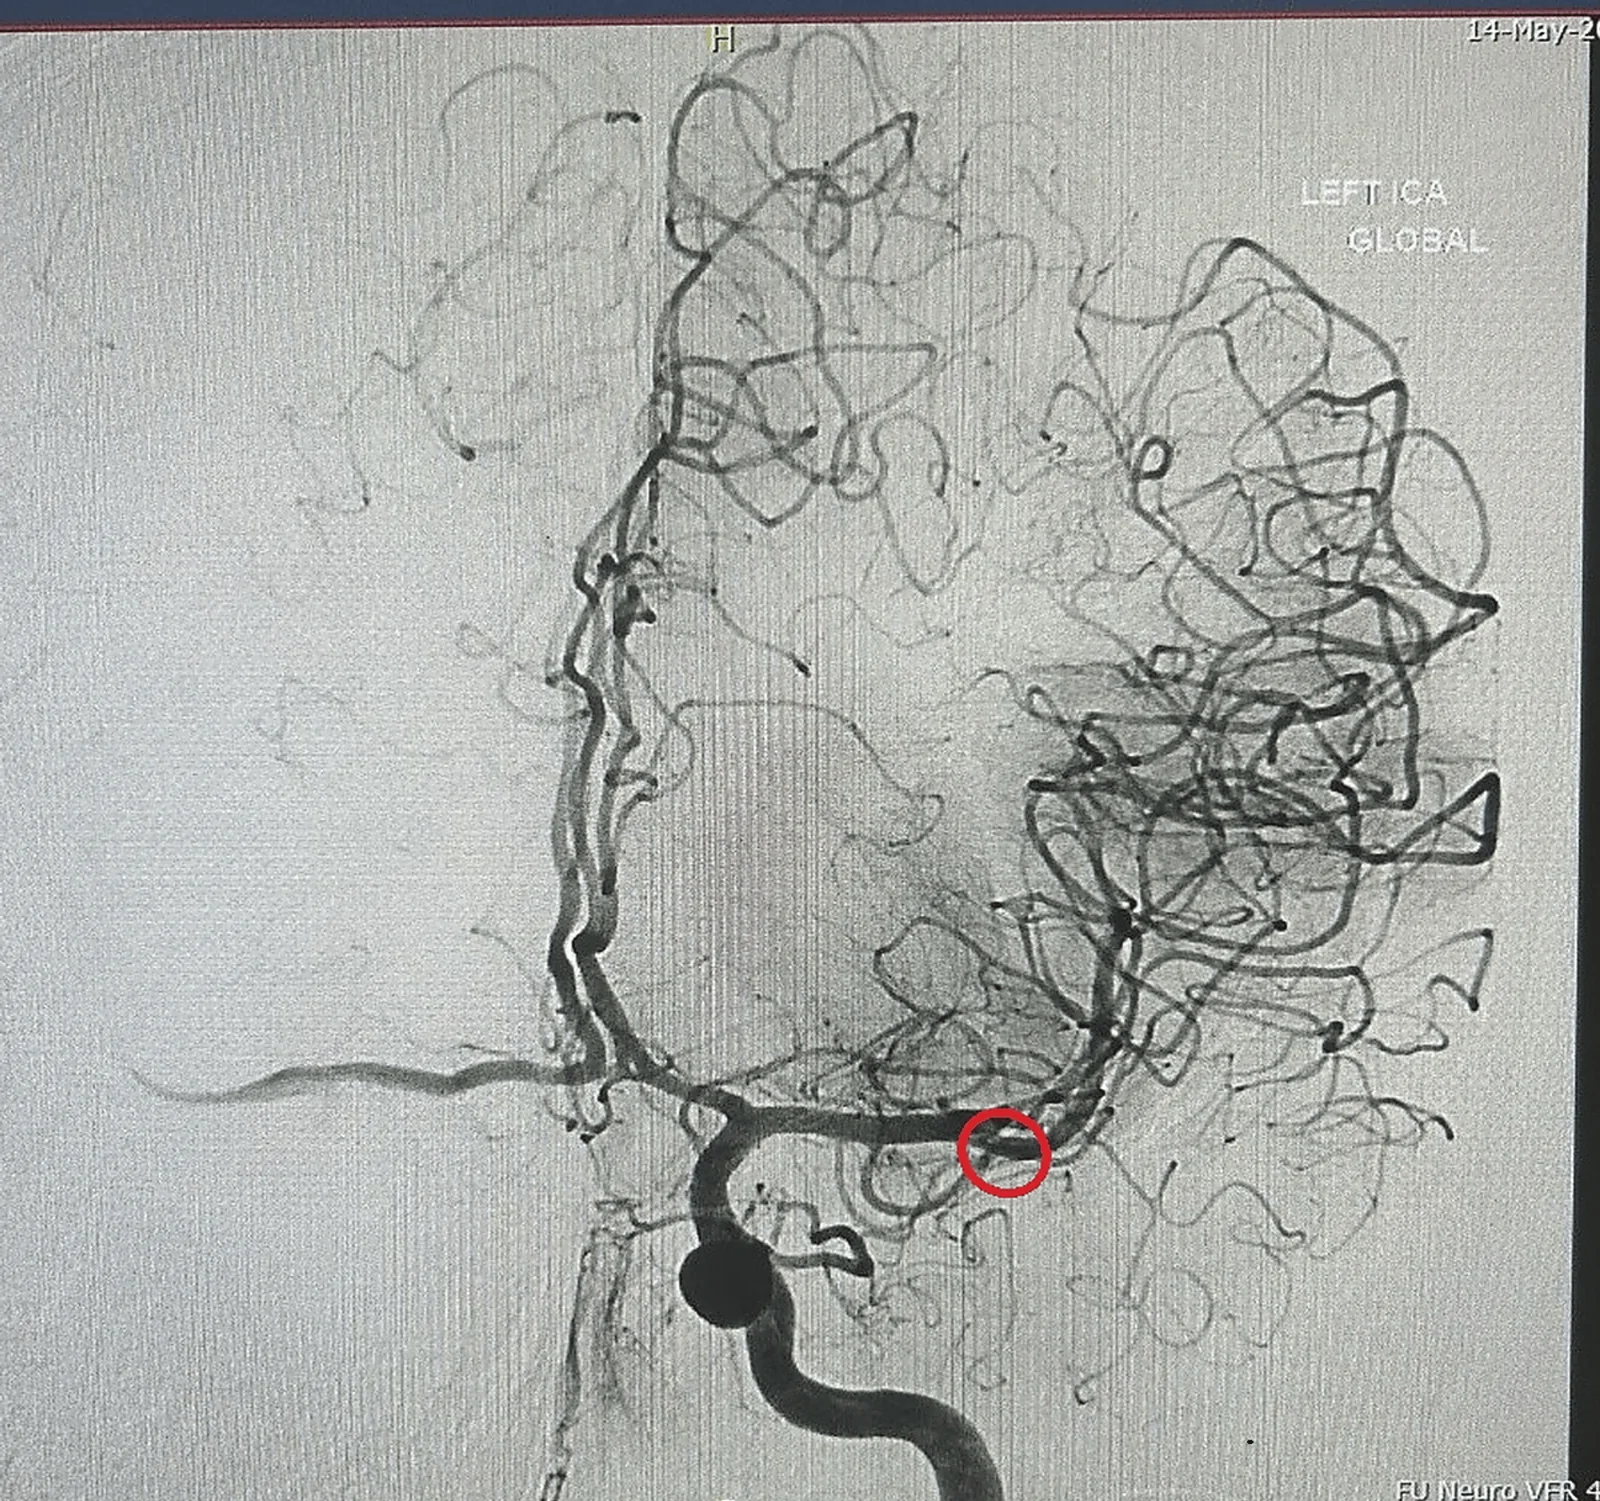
Post thrombolysis and thrombectomy CT cerebral angiography Cerebral angiography following thrombolysis and initial mechanical thrombectomy demonstrating successful reperfusion of the left middle cerebral artery (circled).
